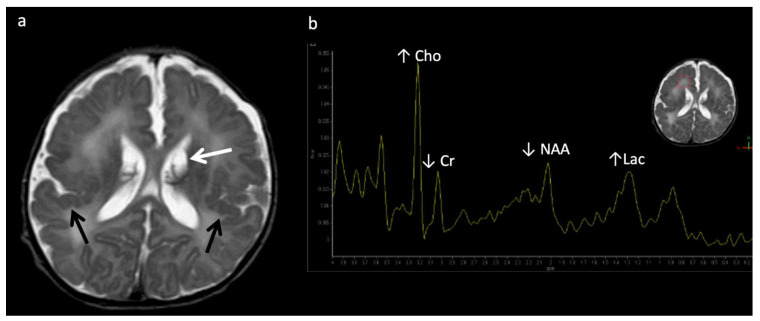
Zellweger syndrome in a 4-day-old full-term infant with multiple congenital anomalies, hypotonia, and weak/absent reflexes. (a) Axial T2WI shows findings typical of Zellweger syndrome, including bilateral perisylvian polymicrogyria (black arrows), germinolytic cysts (white arrow), and abnormal white matter, which may represent areas of hypomyelination. (b) SV-MRS of the white matter shows elevated Lac (consistent with anaerobic metabolism and indicating active on chronic disease, given MRI appearance), elevated Cho, and depleted NAA and Cr (compatible with neuronal/axonal damage/loss).
